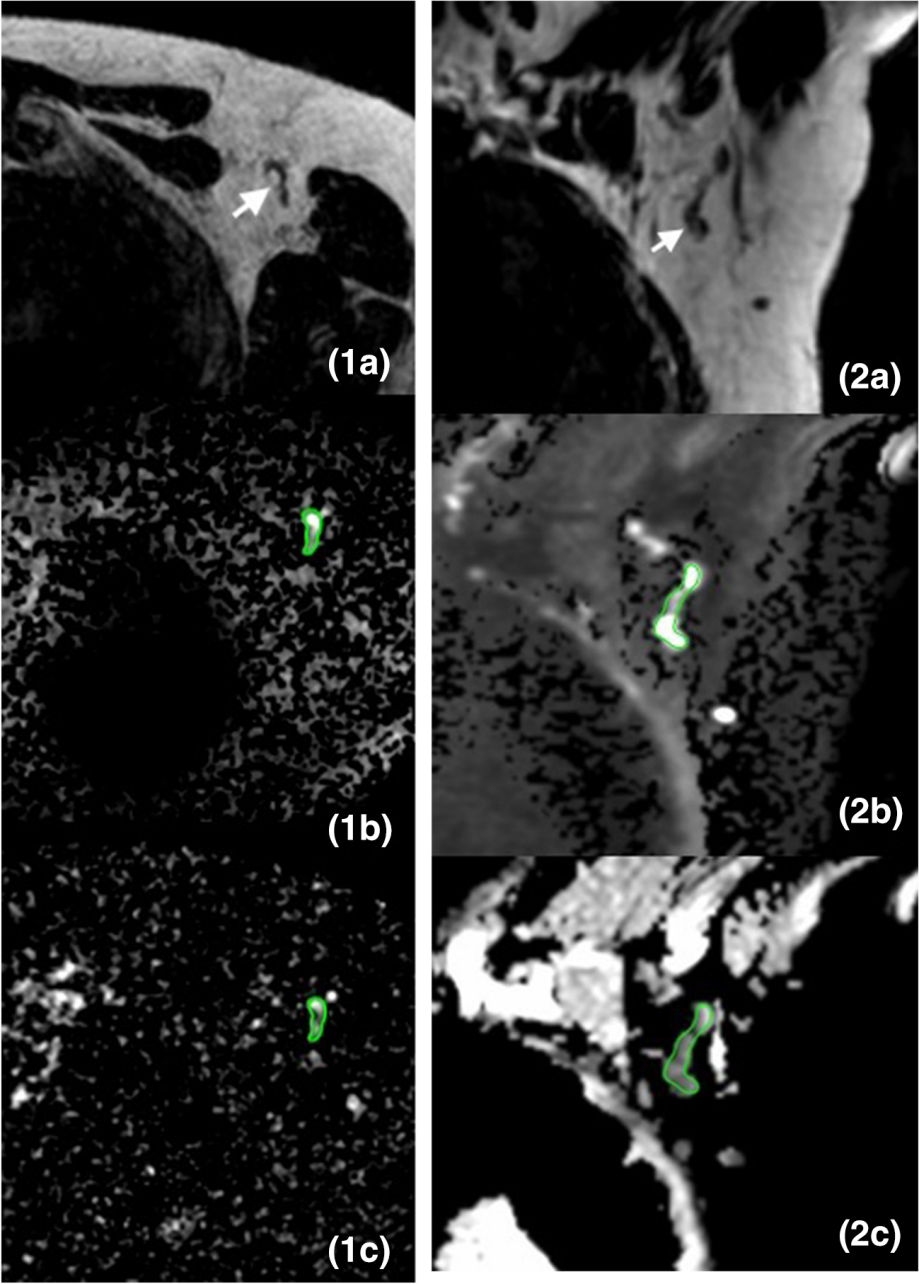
Images of a 24-mm large invasive ductal carcinoma in the left breast of a 76-year-old female patient, which was treated with breast-conserving surgery and SLNB (pT2N0). The white arrow points to an axillary lymph node. The green delineation shows an axillary lymph node. 1a Axial T2W breast MR image shows the axillary lymph node with the longest axis. 1b Axial DW breast MR image (*b*-value = 800 s/mm^2^) shows the same axillary lymph node with relatively high signal intensity. 1c Axial ADC map of breast MRI shows corresponding lymph node with relatively high signal intensity with an ADC value of 0.526 × 10^−3^ mm^2^/s. 2a Coronal T2W dedicated axillary MR image shows the axillary lymph node with the longest axis. 2b Coronal DW dedicated axillary MR image (*b*-value = 800 s/mm^2^) shows the same lymph node with relatively high signal intensity. 2c Coronal ADC map of dedicated axillary MRI shows corresponding lymph node with relatively low signal intensity with an ADC value of 0.940 × 10^−3^ mm^2^/s
